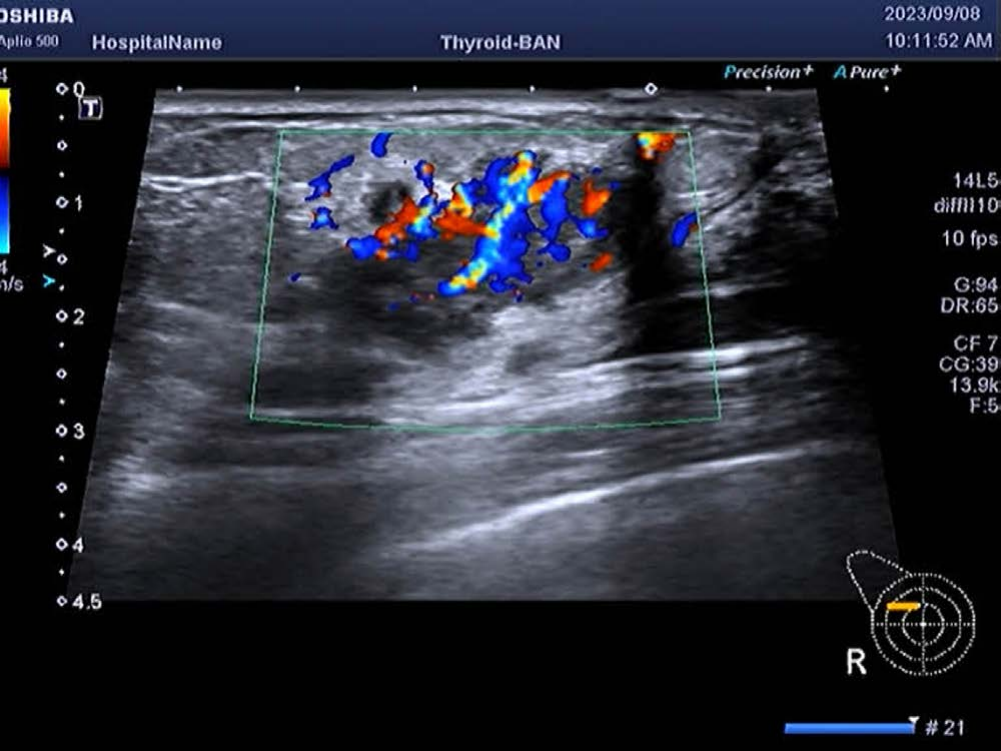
The breast ultrasound of the patient indicated that there was an abnormal nodule in the right breast, which was rich in blood flow signals, and breast cancer was more likely.
